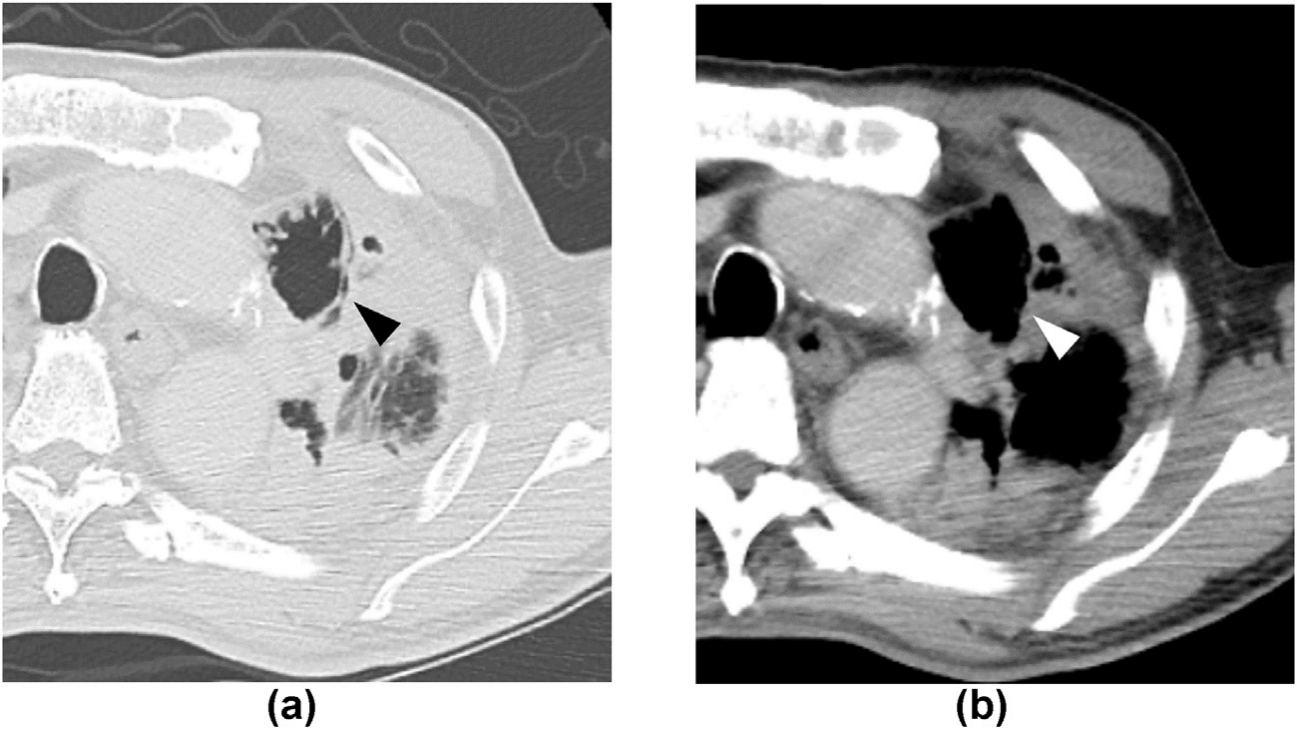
CT image after 6 months of treatment. The lesion has shrunk relative to the size on the pretreatment images. Cavitation is apparent with no signs of infection (A, B, arrowheads).
